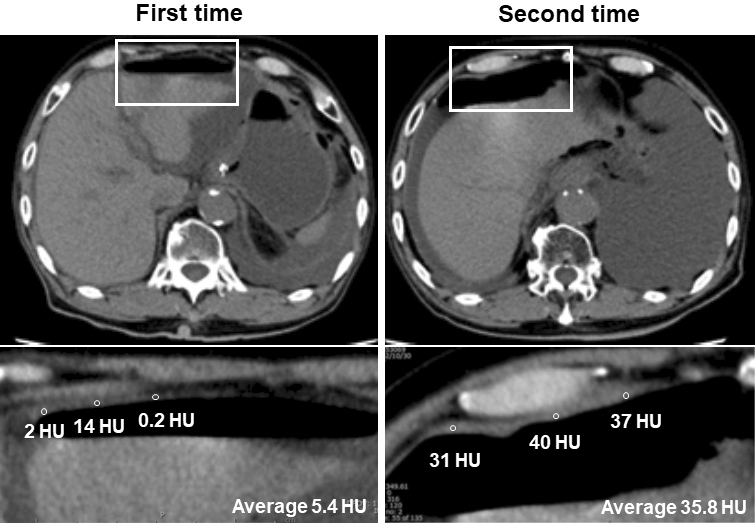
Representative abdominal plain CT images (upper panels) and peritoneal CT attenuation values (lower panels) in a patient with two duodenal perforations. The region of interest was created, and three arbitrary points on the peritoneum adjacent to free air were measured. Peritoneal CT levels (HU) were inserted. CT: computed tomography; HU: Hounsfield units.
